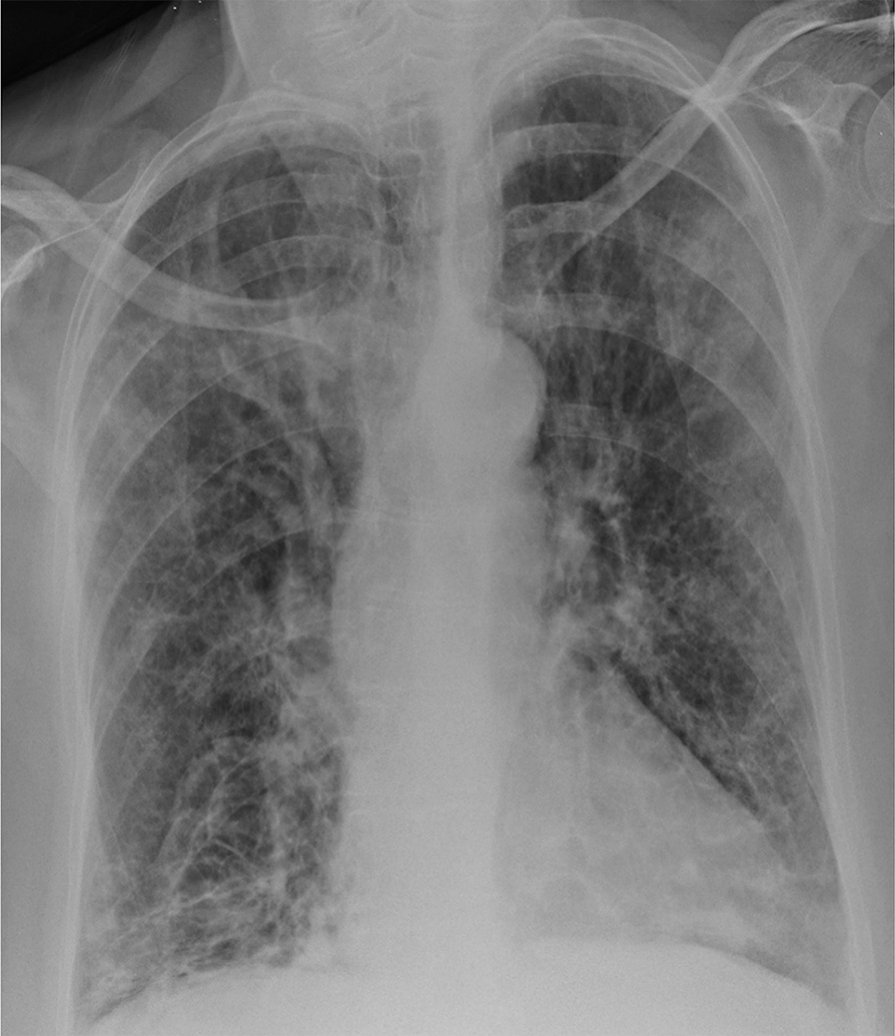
Posteroanterior chest radiograph performed acutely demonstrating hyperexpansion, ring and tram line shadows, coarse lung markings and architectural distortion. No new changes were seen compared with previous radiographs.
